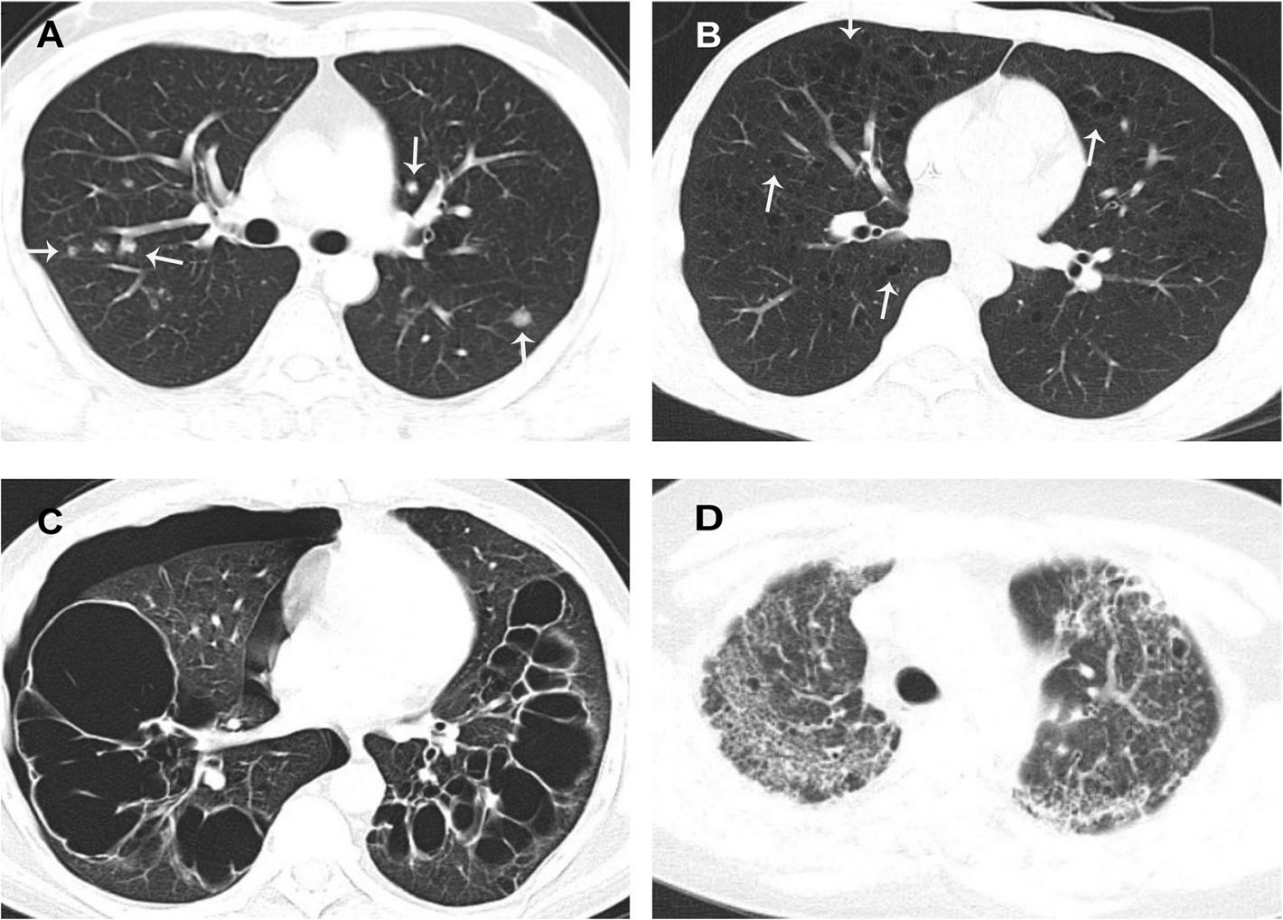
HRCT imaging findings of adult LCH patients with pulmonary involvement: (a) multiple modules (white arrows), (b) thin-walled cysts (white arrows) and emphysema, (c) pulmonary bulla, and (d) honeycomb and reticular patterns
